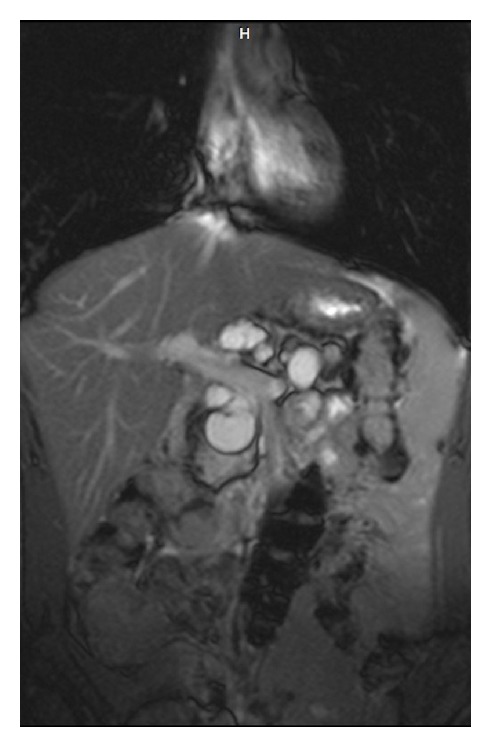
T2-weighted coronal MR image—TRUFI (True Fast Imaging with steady-state-free precession) sequence—obtained at the level of the portal-splenic venous confluence shows multiple high signal cysts of varying size replacing the pancreas.
